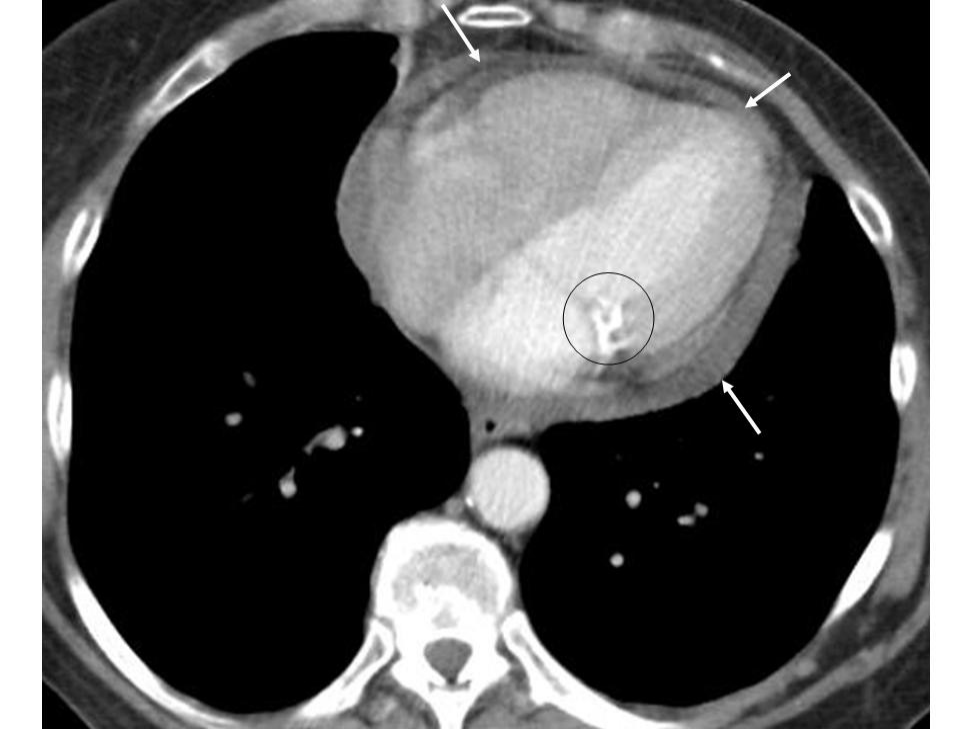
Contrast enhanced chest CT of a 65 years old female shows accumulation of fluid around the heart (arrows) suggesting pericardial effusion. Given that she was a known case of carcinoma breast, pericardial effusion was considered to be malignant in nature. Mitral valve calcification (circle) is noted at the junction of the left atrium and left ventricle
